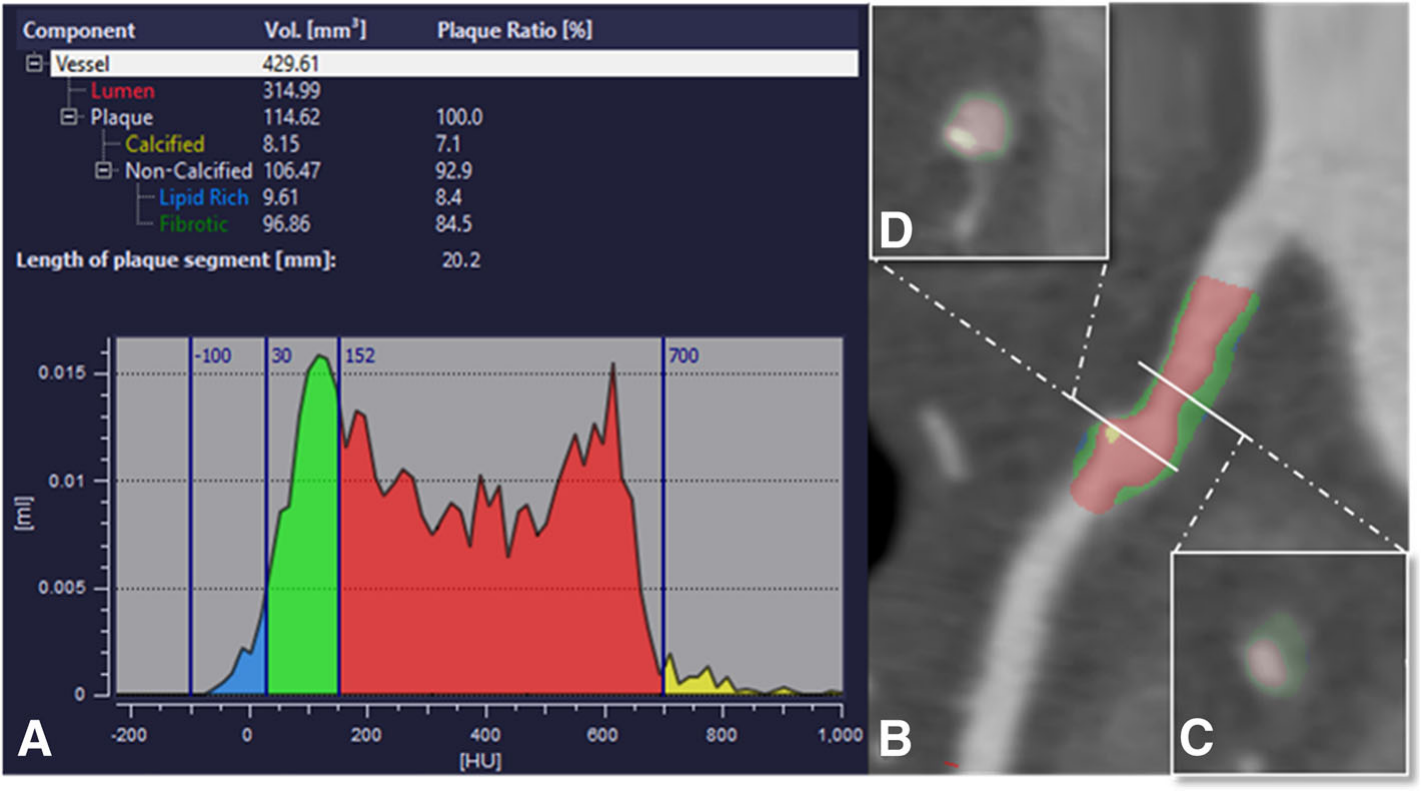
Coronary plaque analysis from computed tomography coronary angiography. To obtain quantitative and qualitative patient-specific plaque markers, the plaque burden is analyzed according to tissue attenuation based on Hounsfield Units (a). Example of the longitudinal tracking by an automatically generated curved multiplanar image data reformation along the vessel centerline (b) and corresponding transverse luminal sections perpendicular to the centerline (c and d) provided by the software
